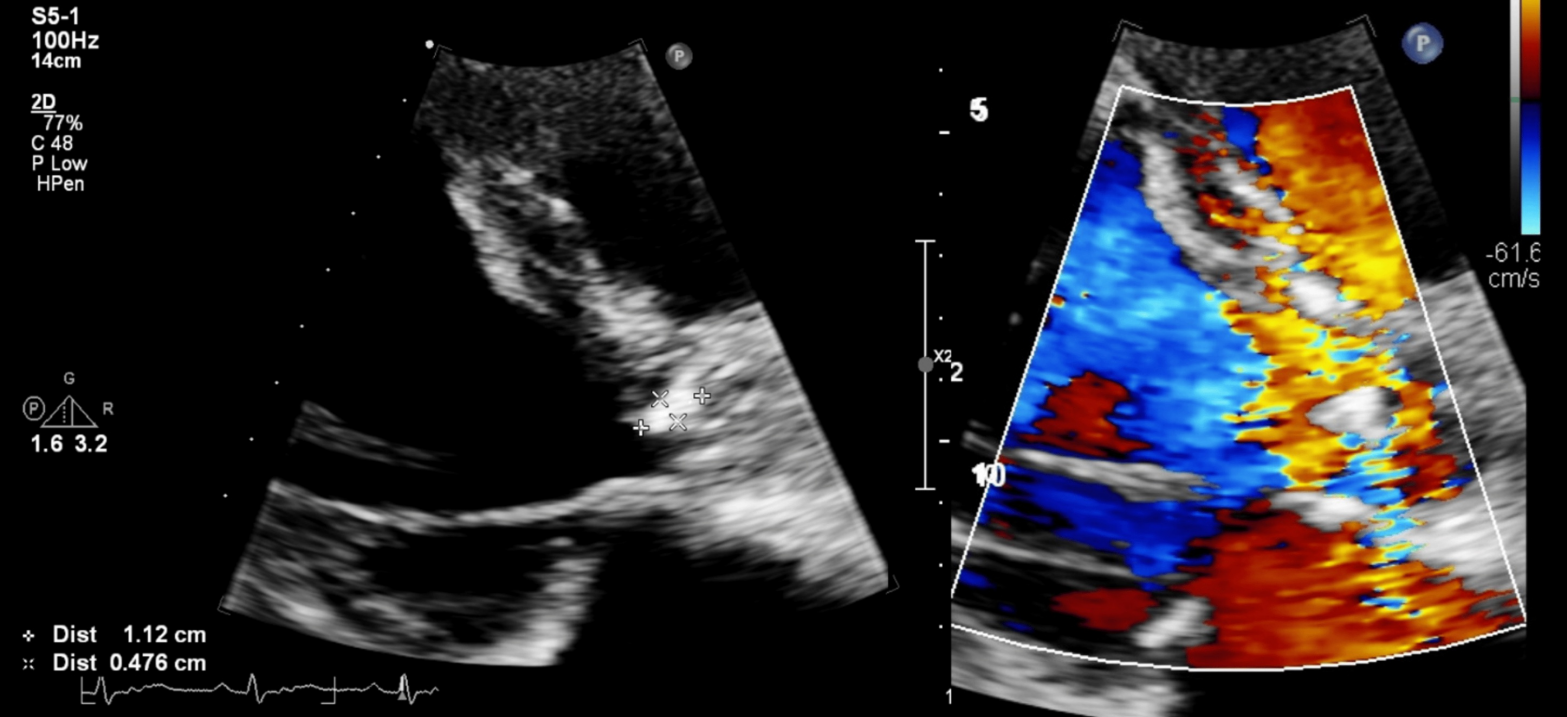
Echocardiogram showing aortic valve vegetation and moderate aortic regurgitation.
